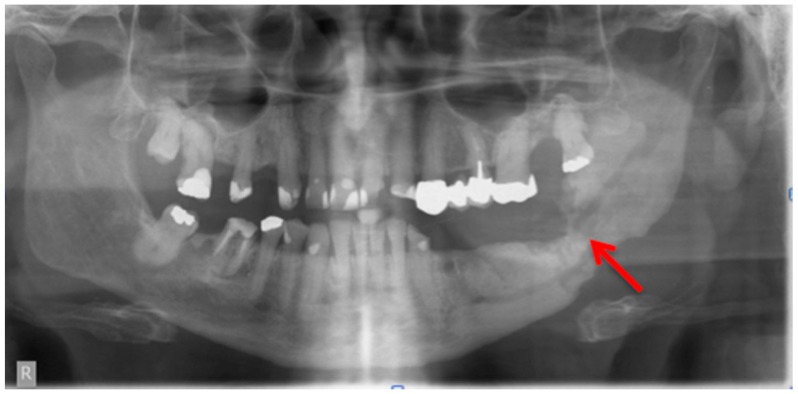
Panoramic radiograph: Patient: 66 years old, male, secondary osteoporosis due to castration, alendronate. Red arrow indicates the almost invisible fracture. The computed tomography (CT) scan is shown in Figure 6 and the corresponding single photon emission computed tomography (SPECT) and SPECT/CT images in Figure 8 and Figure 9 respectively. AAMOS staging: stage 3.
